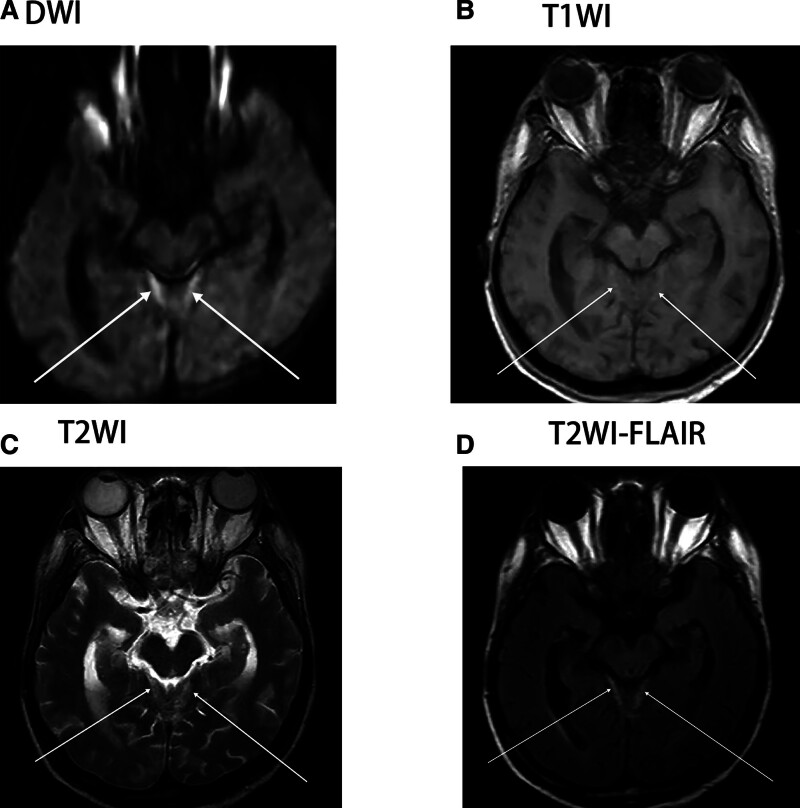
The MRI of the brain. (A–D) Symmetric slightly long T1 and slightly long T2 signals are observed in the area of the tentorium cerebelli near the midline (as indicated by the arrows), showing hyperintensity on FLAIR (fluid-attenuated inversion recovery) and diffusion restriction signal on DWI (diffusion-weighted imaging). DWI = diffusion-weighted imaging, FLAIR = fluid-attenuated inversion recovery, MRI = magnetic resonance imaging.
